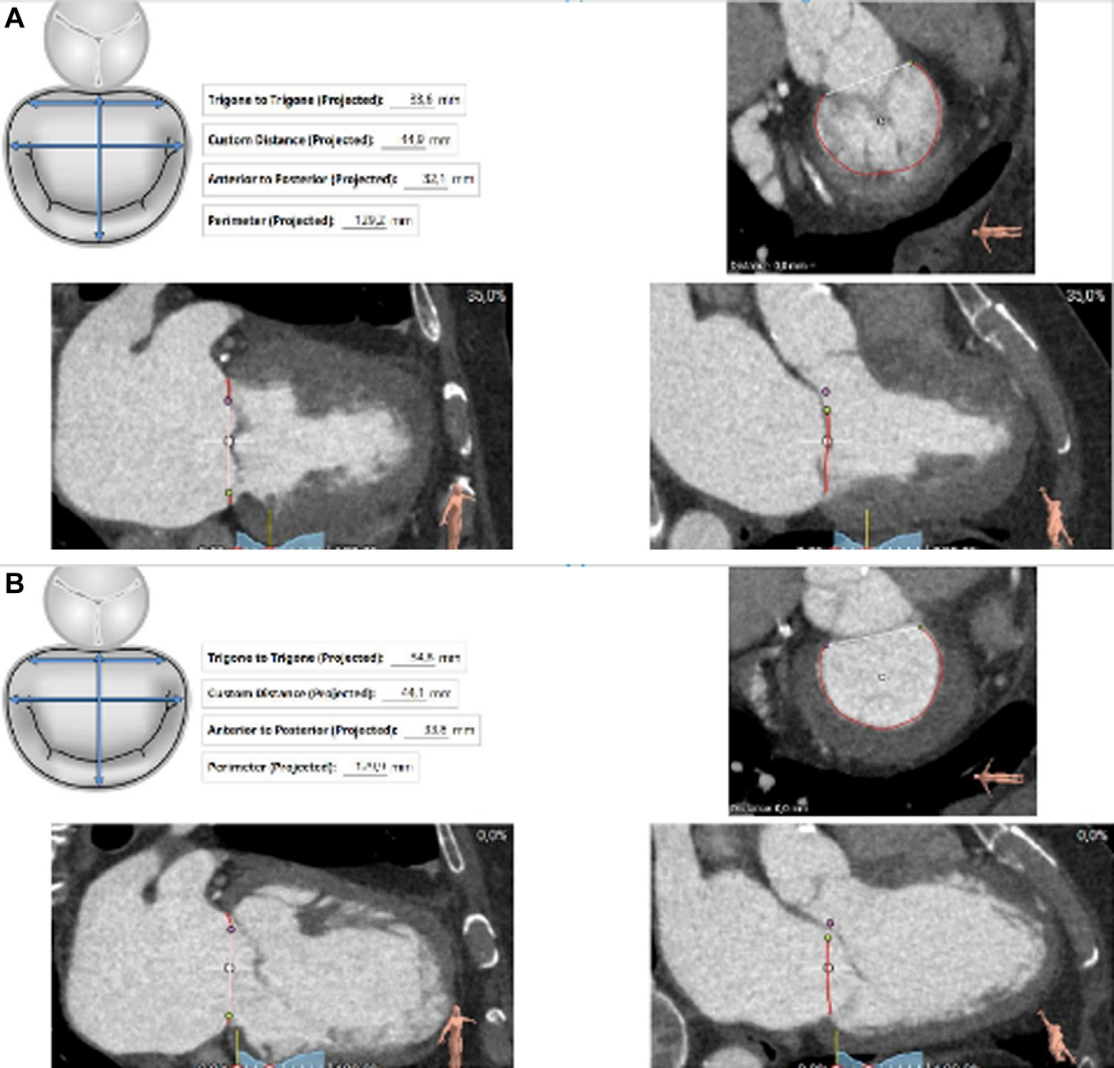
Cardiac Computed Tomography Study of annular segmentation during (A) systole and (B) diastole.
